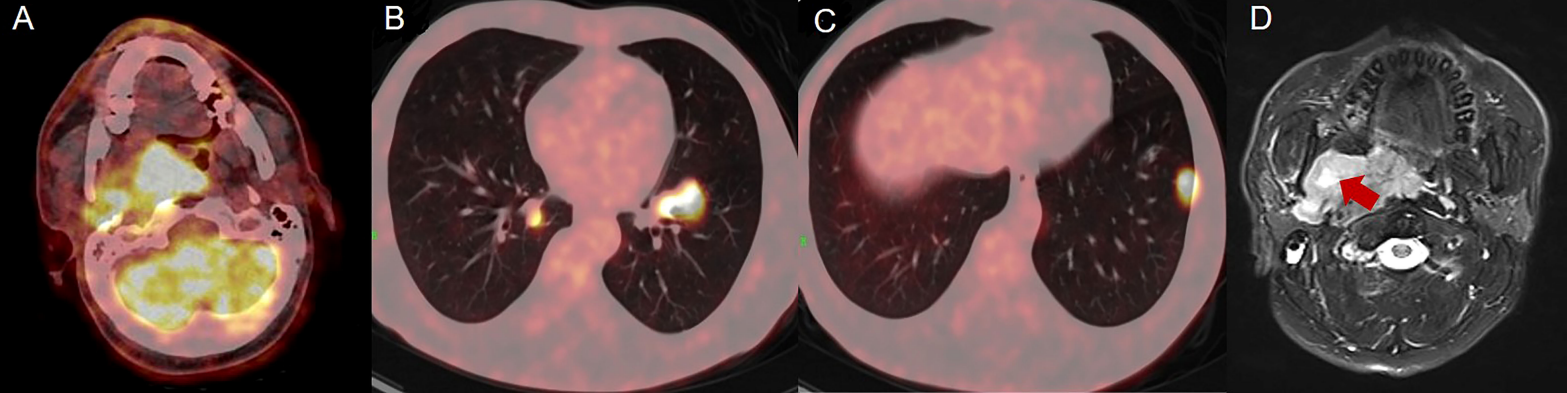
Positron emission tomography and computed tomography (PET/CT) and magnetic resonance images (MRI) of lesion and mass. (A–C) PET/CT revealed a mass in right lateral wall of the pharynx, along with lung metastases. (D) MRI showed a soft tissue mass with a size of 7.5 cm × 7.5 cm × 3 cm.
